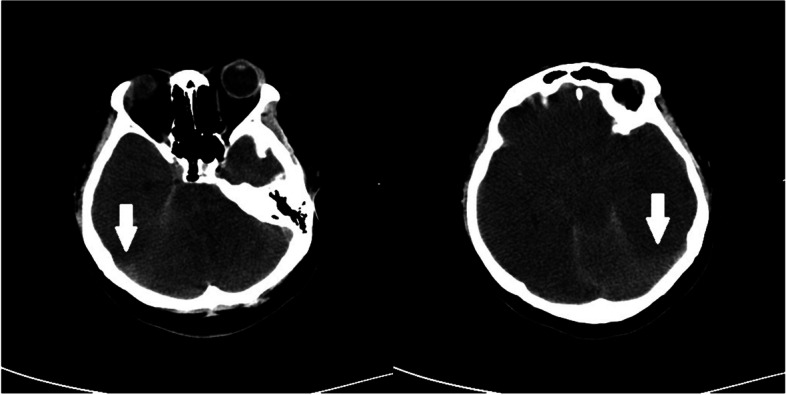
On the fifth day of admission,CT of the head showed that diffuse cerebral edema
